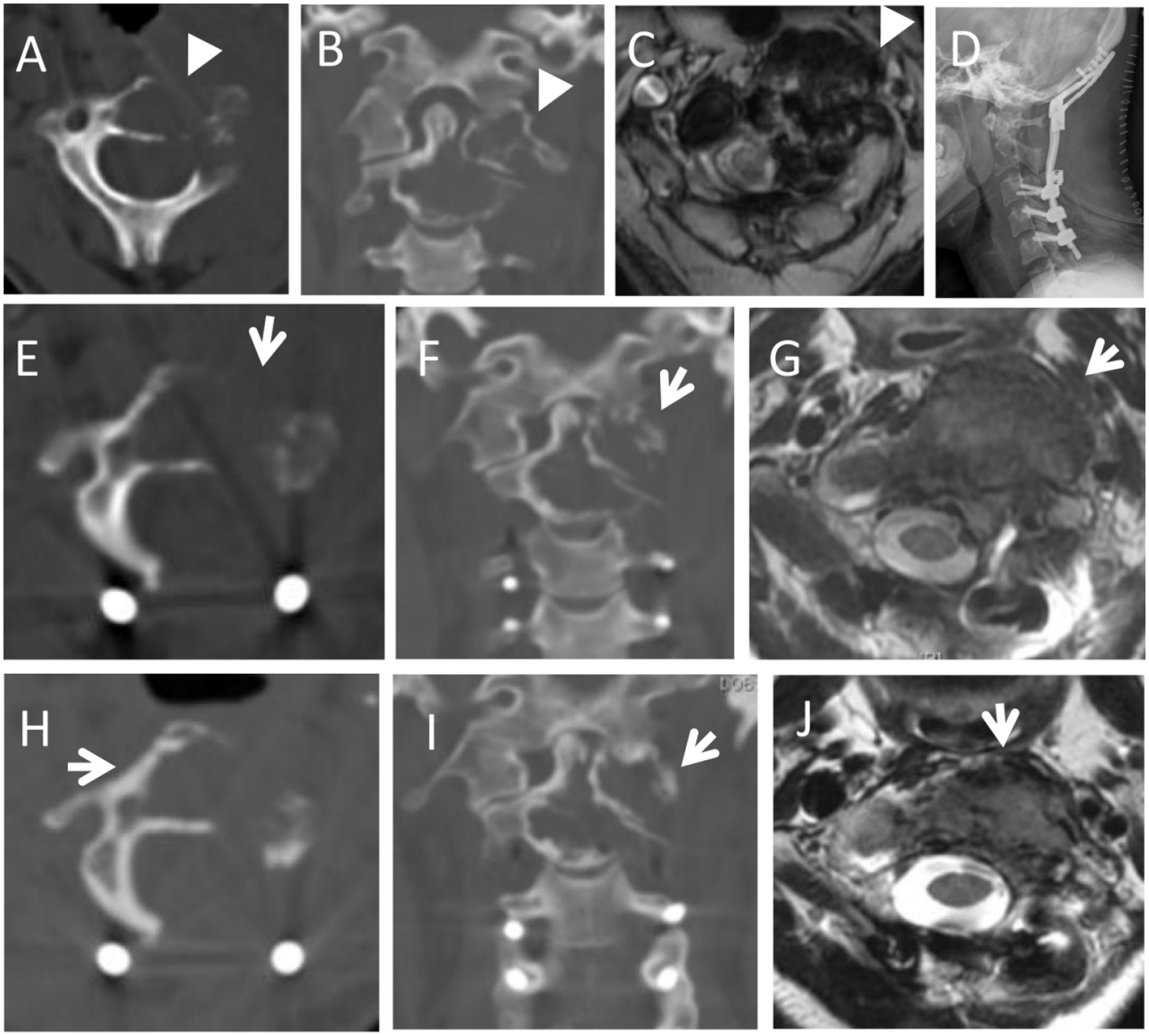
A 32-year-old female with neck pain for four months, and worsened in the following 2 months. Preoperative imaging examination showed an osteolytic lesion was located in the C1-2 (arrowheads in A, B). She underwent STR because of lesion adhesion to the vertebral artery. In 3-month follow-up, CT and MRI scans revealed local progression with soft tissue mass (arrows in E,F,G). She underwent radiotherapy. And at the 69-month follow-up, the lesion was stable and the volume of soft tissue mass was reduced with slight new bone around the osteolytic lesion (arrows in H,I,J). (A,B): Preoperative axial and coronal CT scans; (C): T2-weighted axial MRI scans; (D): Postoperative X-ray; (E,F): Axial and coronary CT scans in 3rd month after surgery; (G): T2-weighted axial MRI scan at the 3-month follow-up after surgery; (H–J): Axial and coronary CT scans, and T2-weighted axial MRI scan at the 69-month follow-up after radiotherapy.
